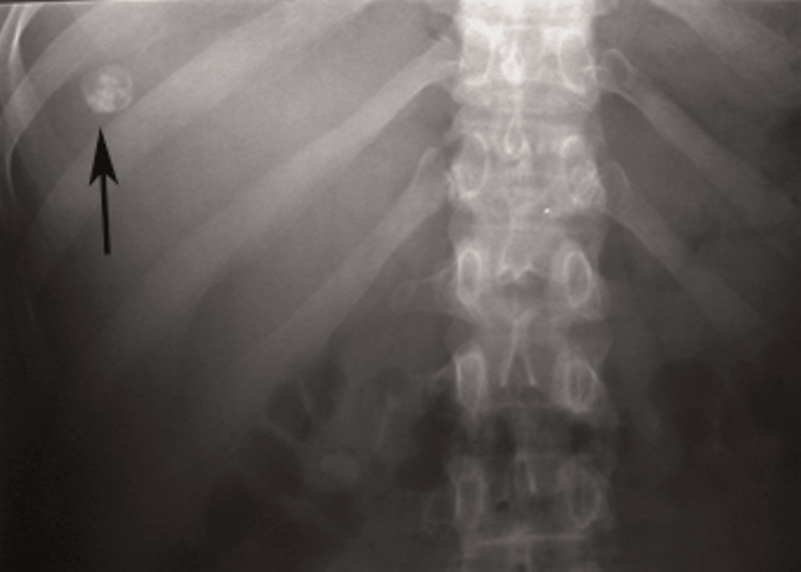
Plain film of the right hypochondrium shows a well defined, rounded calcification with an inner snowflake pattern.
